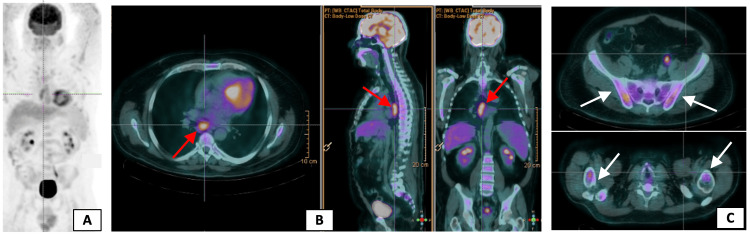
Whole-body FDG-18 PET-CT scan (A) showing a diffusely increased uptake in mediastinal lymphadenopathy (B, red arrows) and skeleton (C, white arrows) FDG-18: fludeoxyglucose-18; PET: positron-emission tomography; CT: computed tomography
